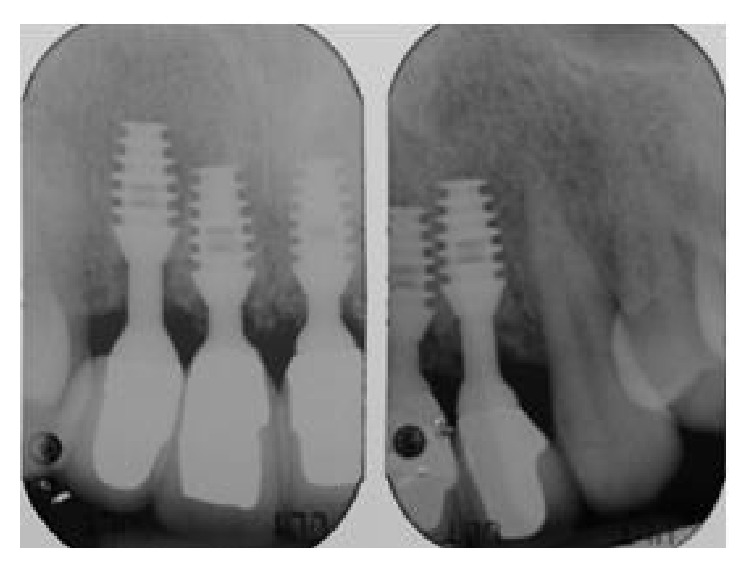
Radiographs at five-year follow-up showing interproximal bone and bone at implant shoulder.
